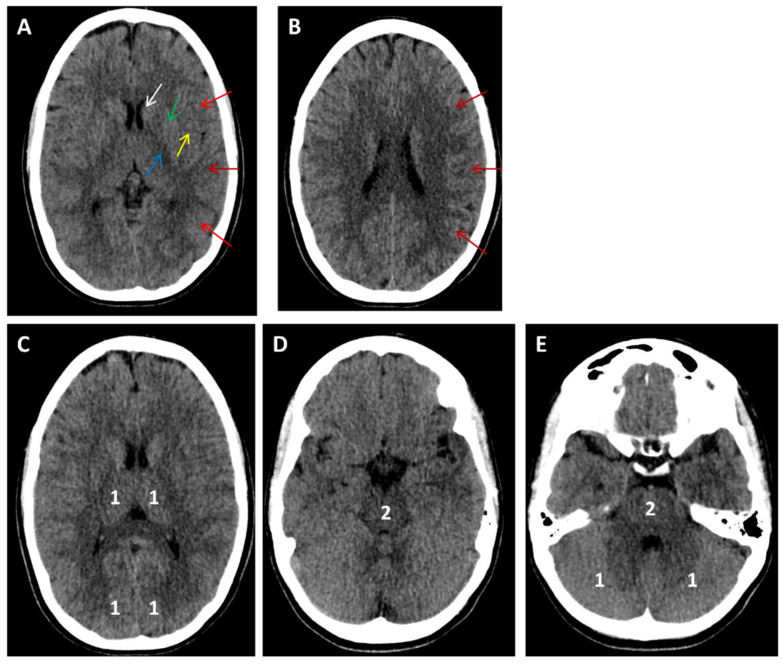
Alberta stroke program early CT score (ASPECTS; shown in panels (A,B)) and posterior circulation ASPECTS (pc-ASPECTS; shown in panels (C–E). (A): Regions assessed for early ischemic changes in ASPECTS at the ganglionic level shown with arrows (white: caudate; green: lentiform nucleus; yellow: insula; blue: internal capsule; red arrows: M1, M2, and M3 MCA cortex). Regions assessed for early ischemic changes in ASPECTS at the supraganglionic level shown with red arrows (M4, M5, and M6 MCA cortex). One point is subtracted for each region. (C–E): Regions assessed for early ischemic changes in the pc-ASPECTS. Numbers indicate the points subtracted for each region.
